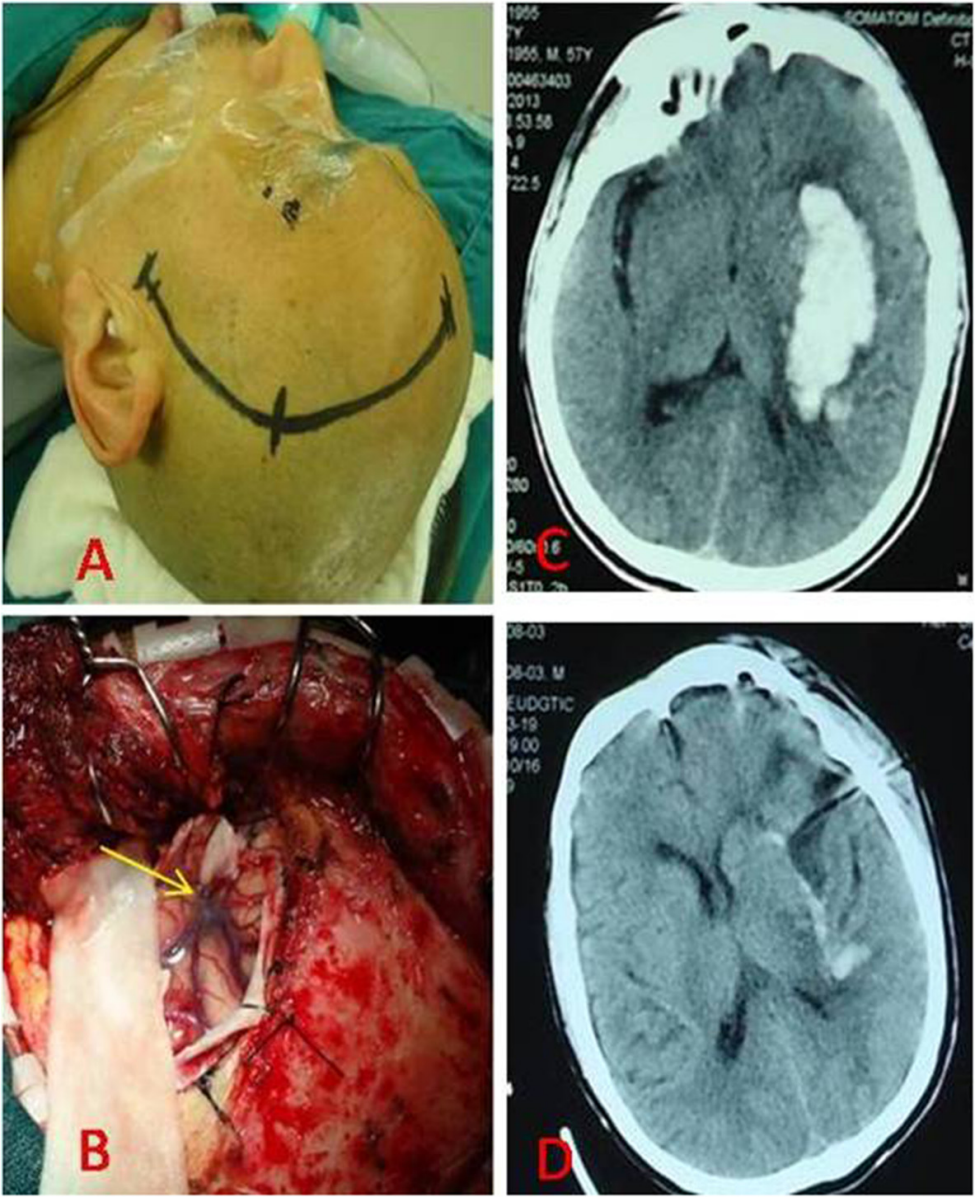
The basal ganglia hematoma was evacuated by transsylvian-transinsular approach. A: A small question mark incision line for the frontal-temporal craniotomy; B: The dural was opened, the sylvian was seen (yellow arrow); C: The CT scan demonstrated a large hematoma within the left basal ganglia of the head, when the patient was admission to neurosurgery department; D: postoperative CT scan showed the intracranial hematoma was totally removed and there was no obvious parenchymal injuries.
